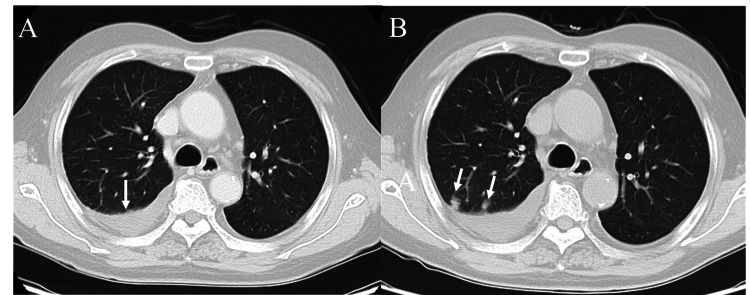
Chest CT scans before and after the development of pulmonary lesions (A) The initial chest CT scan demonstrates a right-sided pleural effusion (white arrow) without any evident lesions in the lung parenchyma. (B) A follow-up chest CT scan performed before the planned surgical pleural biopsy revealed the development of multiple new nodular opacities (white arrows) in the right upper lobe, suggesting an underlying infectious or malignant process.
